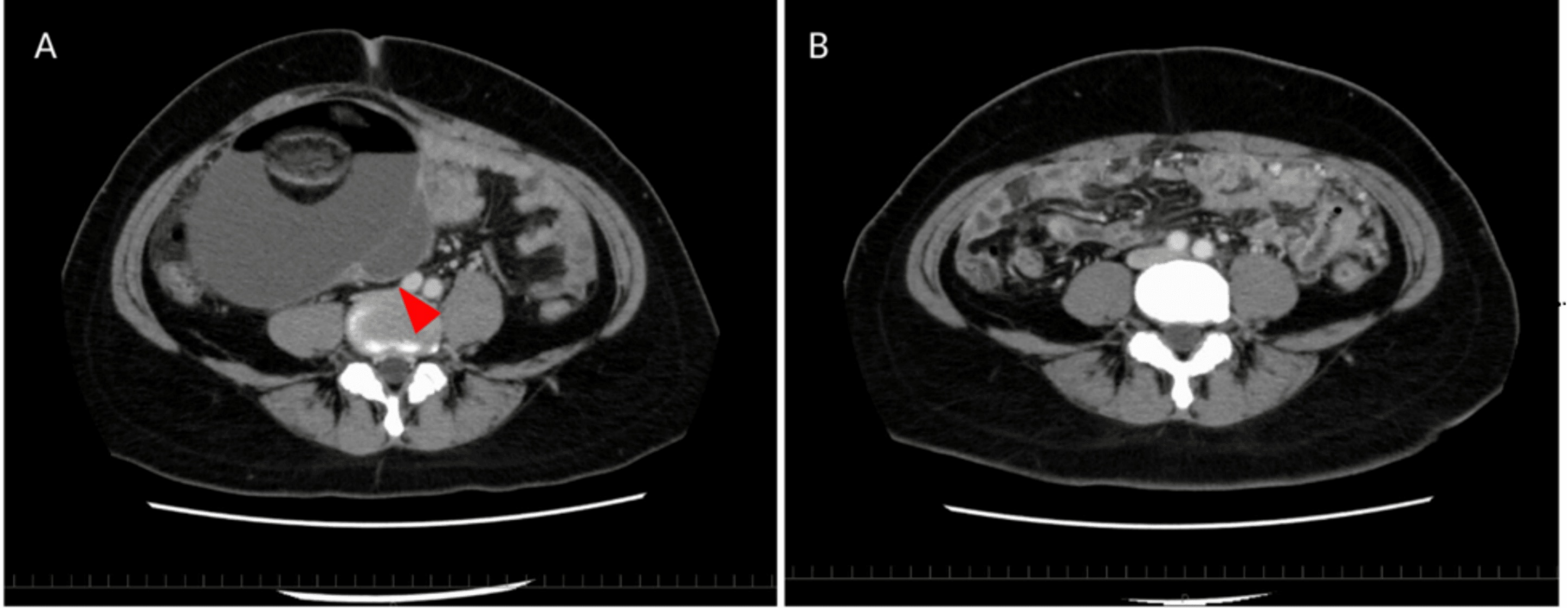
Computed tomography shows peritoneal dissemination and ascites (A) Preoperative CT reveals an ovarian mature teratoma  (red arrowhead) with no evidence of peritoneal dissemination or ascites. (B) Postoperative CT showing peritoneal dissemination and ascites.
